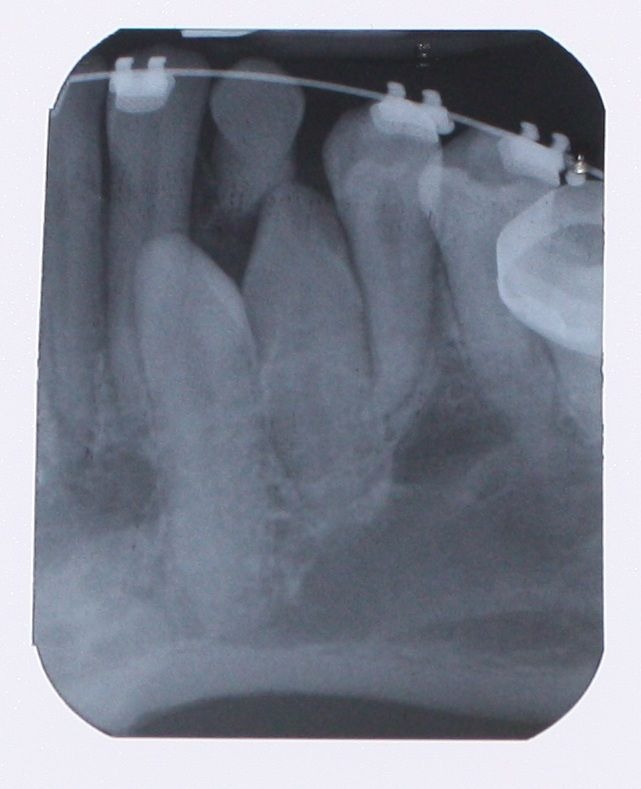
Figure 4. Occlusal view (A) and occlusal radiograph (B) of mandible before treatment, and occlusal radiograph (C) after leveling and aligning.
